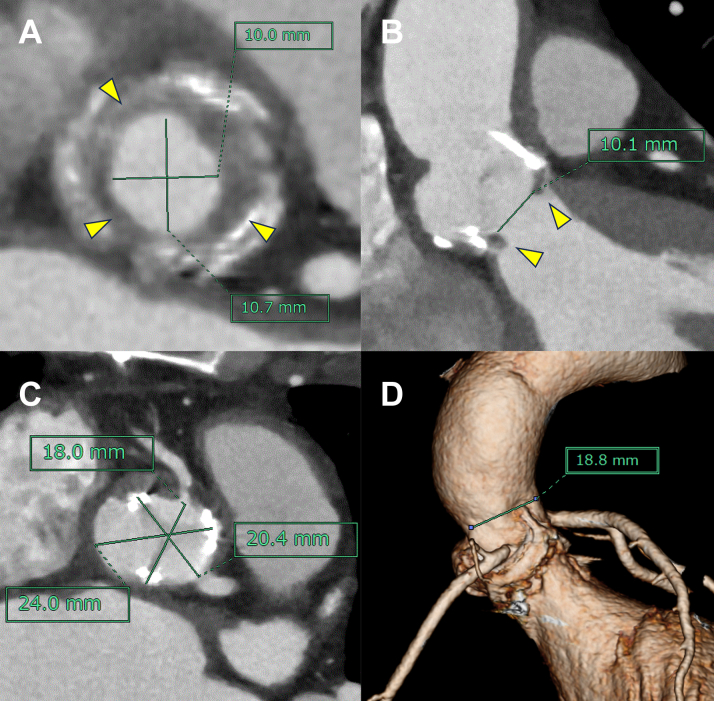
Preoperative Computed Tomography Subvalvular stenosis is shown in the short-axis (A) and long-axis (B) views. The yellow arrowhead indicates pannus. The small root and left ventricular outflow tract are shown in the short-axis view (C) and 3-dimensional reconstruction (D).
